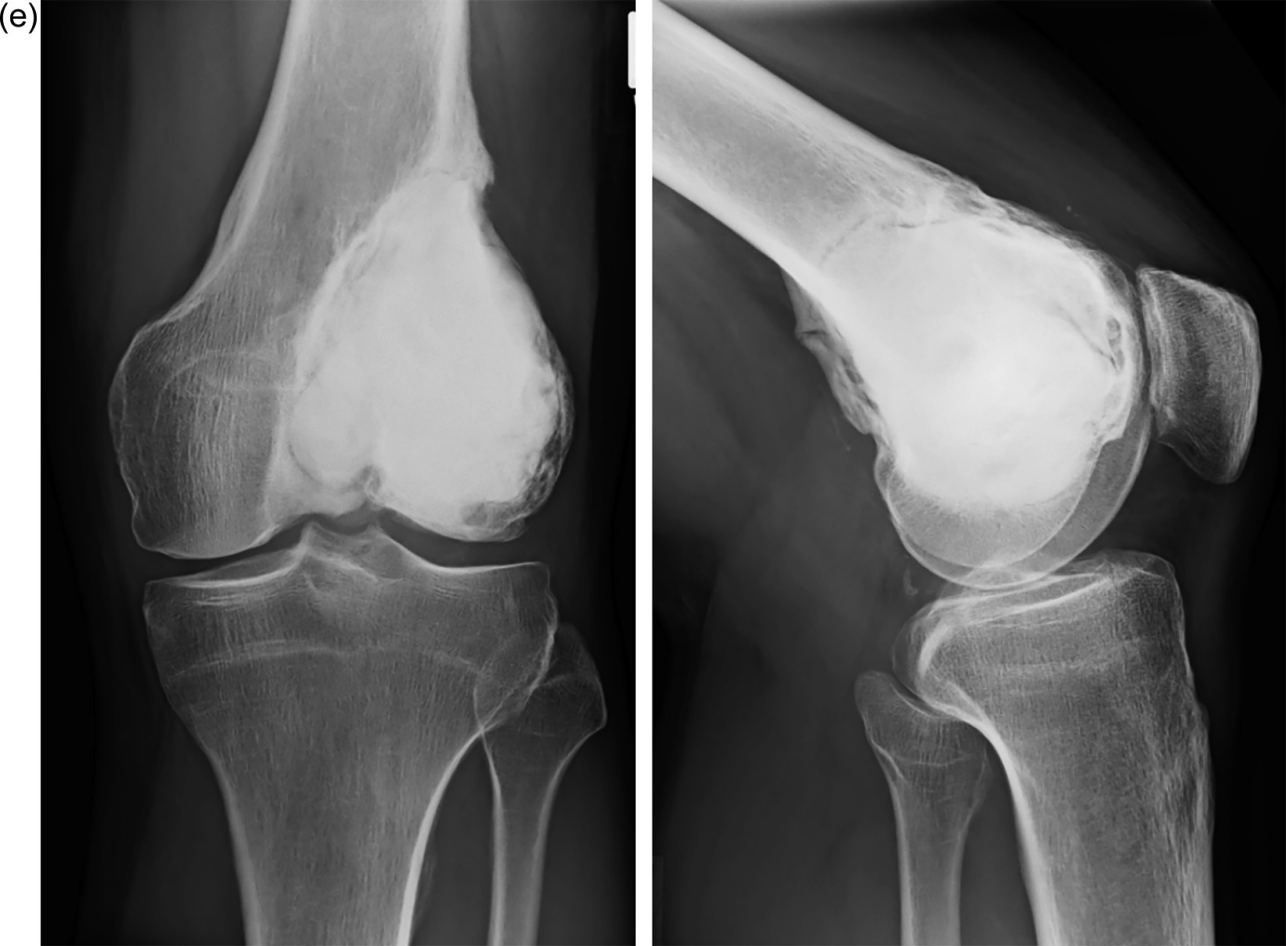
a-e: case of a 36-year-old male patient with GCTB of the distal femur with pathological fracture: 2a: radiographs of the distal femur showing an osteolysis with pathological fracture of the lateral condyle; 2b: CT- (left) and MRI-scan (right) of the distal femur with pathological fracture due to a GCTB; 2c: radiographs 3 months after systemic denosumab treatment showing partial sclerosis of the lesion and consolidation of the fracture, 2d: intraoperative radiograph after intralesional curettage and defect reconstruction with bone cement; 2e: radiographs 36 months after surgery without local recurrence.
